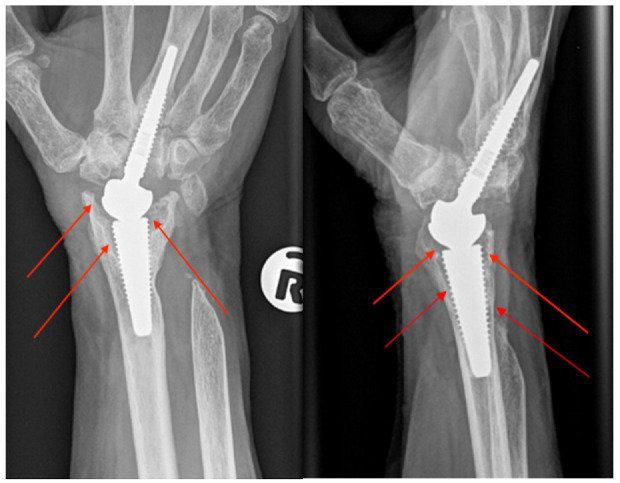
Posteroanterior and lateral X-ray image of the implanted CrN coated MoM Motec TWA. The red arrows show the bone resorption observed around the radius threaded screw which is seen as a progressive black line surrounding the screw, often more noticeable adjacent to the joint.
